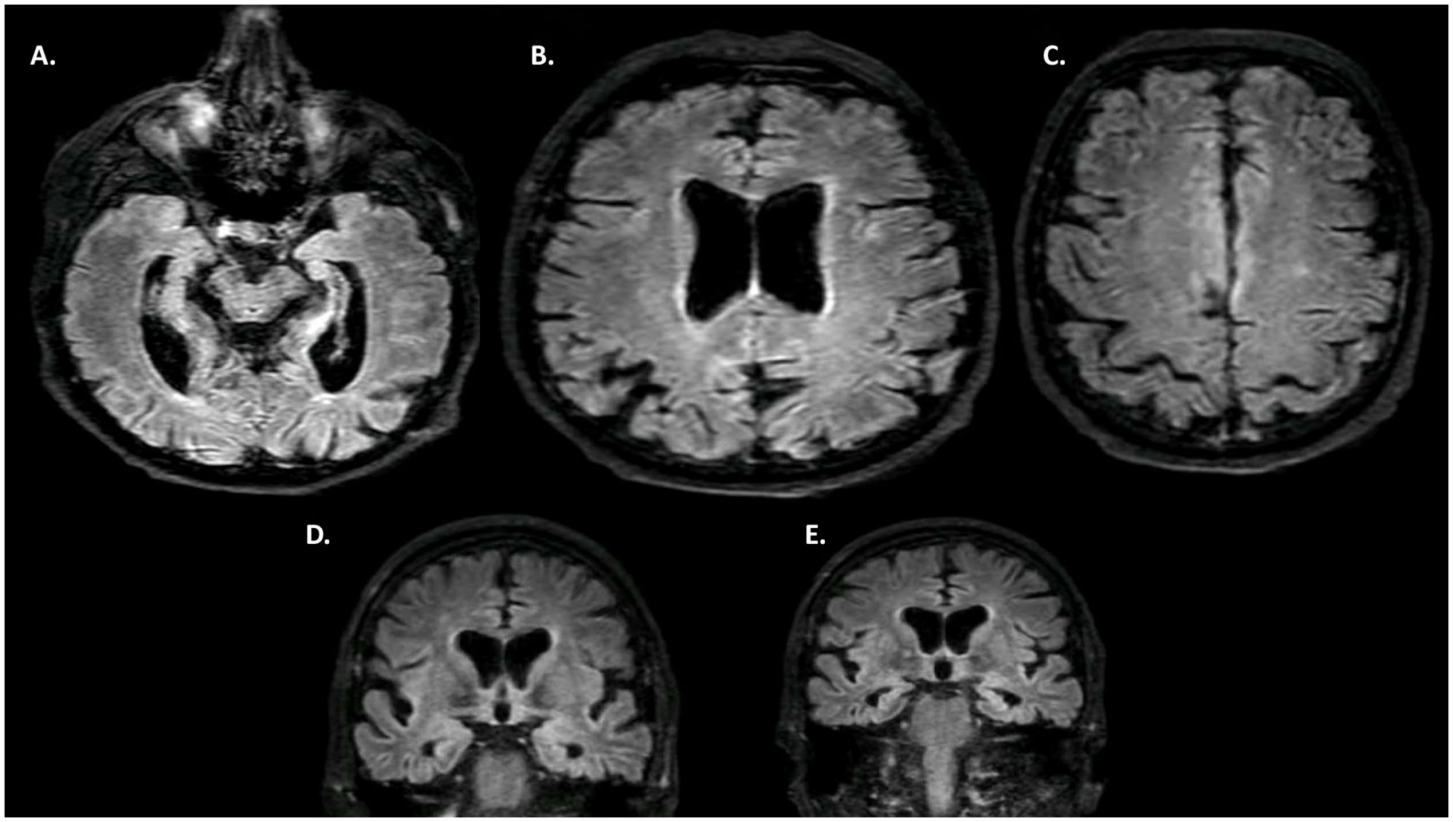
MRI. (A–C) FLAIR axial MRI. (D,E) FLAIR coronal MRI. Diffuse cerebral atrophy, with a marked gradient in the bi-hippocampal regions (D,E—Scheltens 3) and pre-cuneus. Discrete associated vascular leukopathy.
